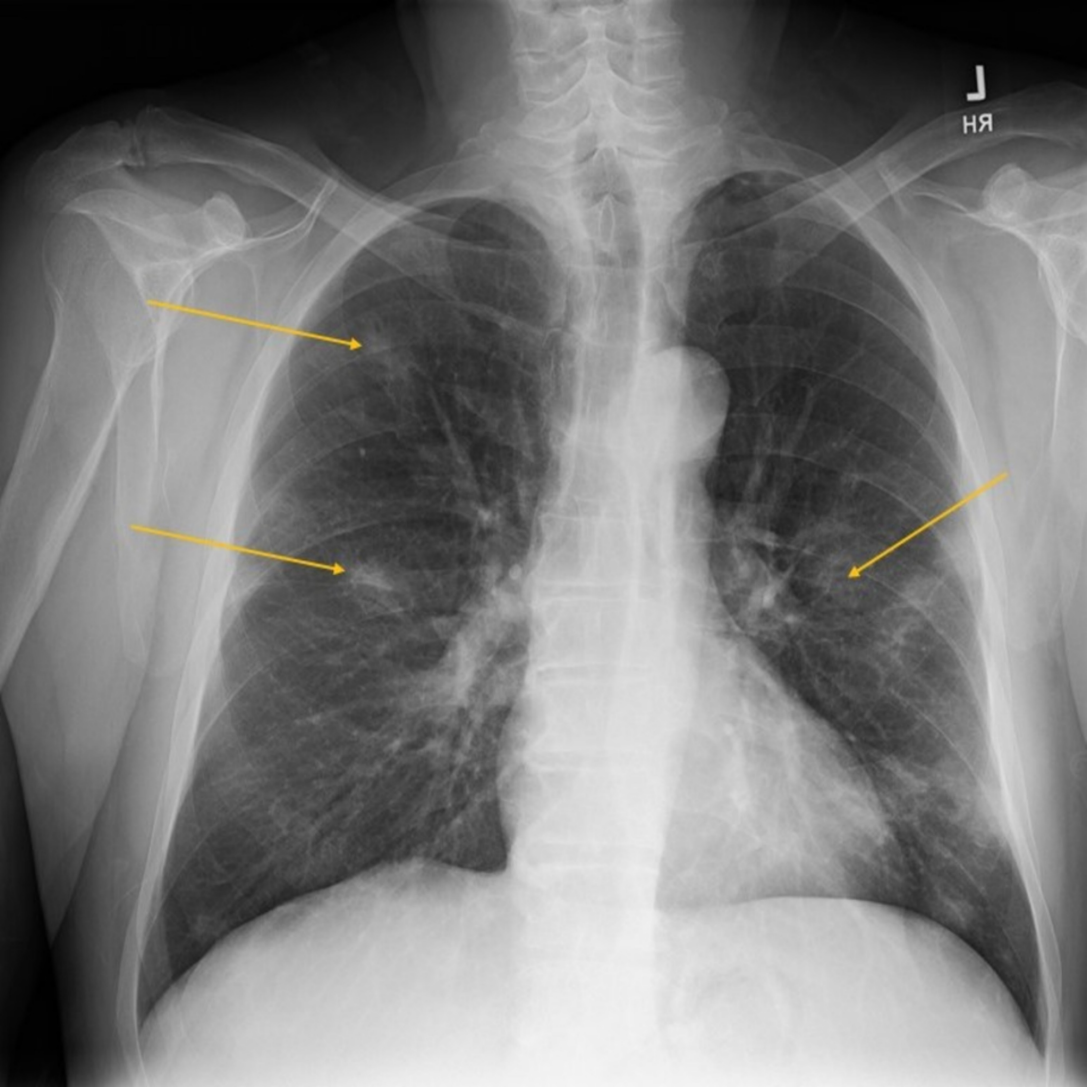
Chest X-ray showing multiple hazy opacities.
